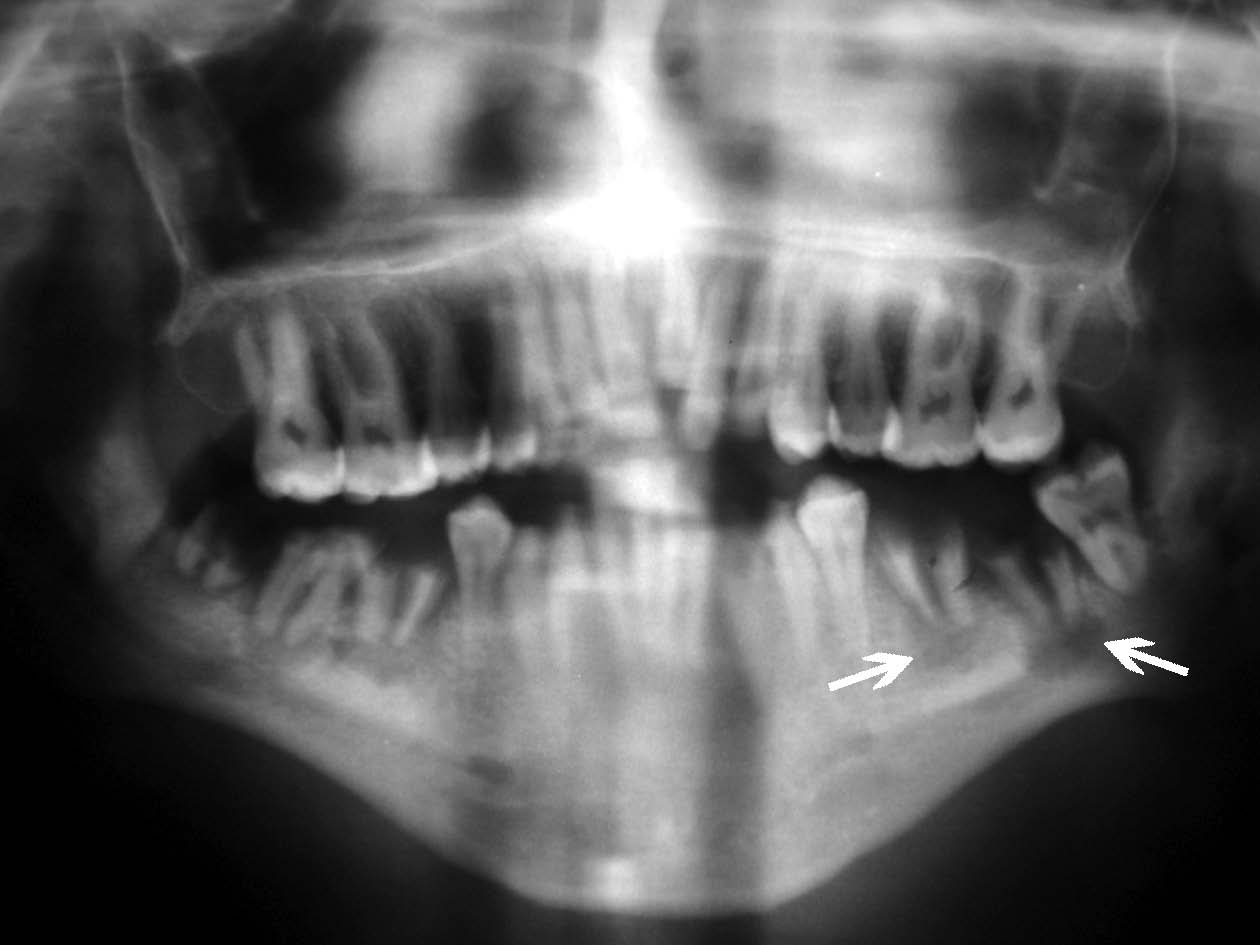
Oral findings in patient A4. A panoramic radiograph showing multiple periapical lesions related to lower molars (arrows).
